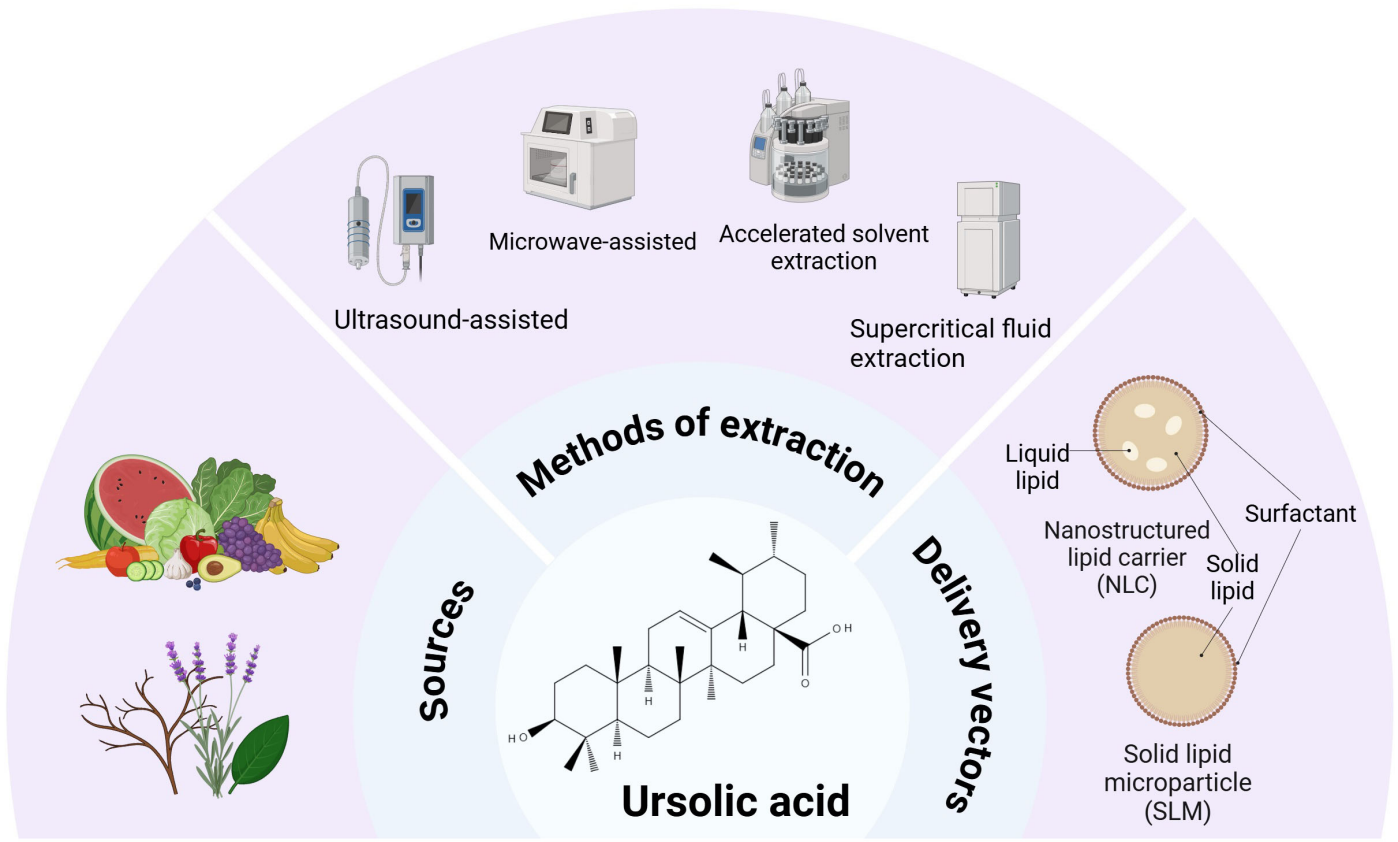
Ursolic acid sources, extraction, and delivery. UA is a pentacyclic triterpenoid compound that is widely distributed in the fruits and roots or leaves of various plants. At present, the main extraction methods for UA are ultrasonic, microwave, accelerated solvent extraction, and supercritical fluid extraction. Two common delivery methods for UA are nanostructured lipid carriers (NLCs) and solid lipid microparticles (SLMs).
